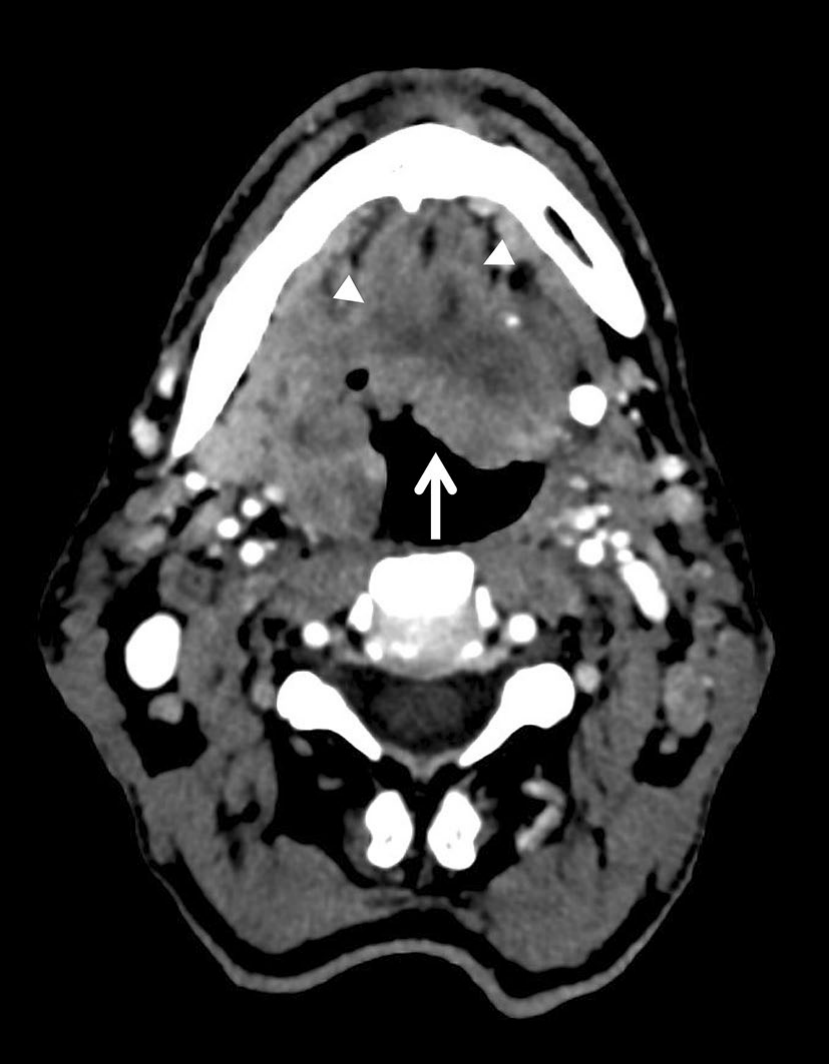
Axial CT slice showing ill-defined contours of a necrotic ulcerated p16− tumour (arrow) of the right palatine tonsil involving the base of the tongue and floor of mouth in a 53-year-old male smoker. Genioglossus muscles were also involved (arrowheads).
